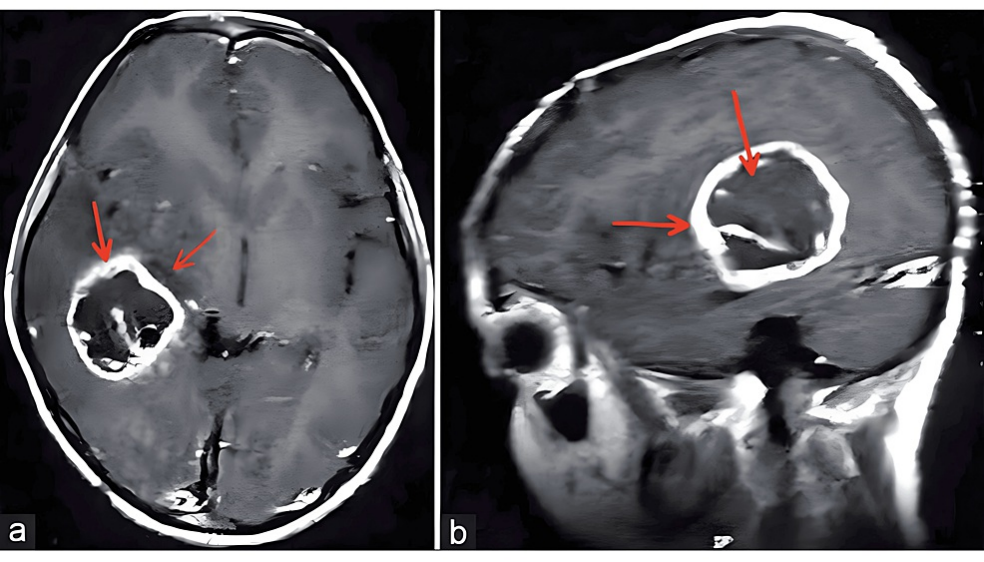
Contrast MRI. Contrast MRI of the brain axial (a) and sagittal images (b) showing a ring-enhancing lesion in the right temporoparietal region with an irregular outline, mass effect, peripheral contrast uptake, and central necrosis.
